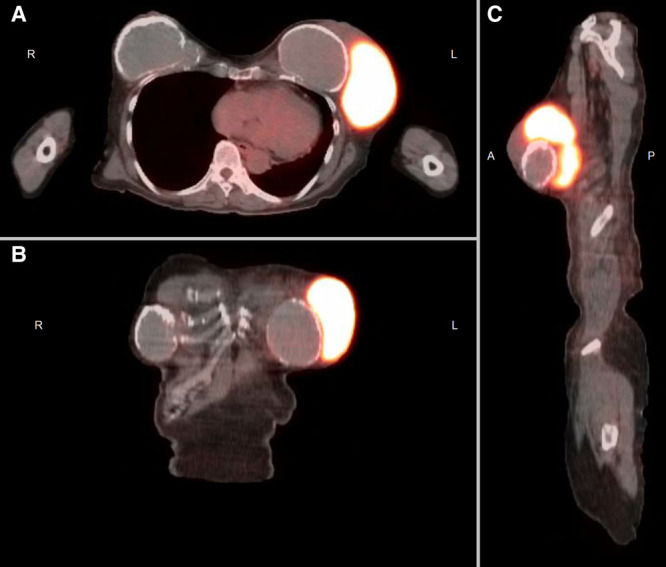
PET-CT imaging demonstrating intensely avid periprosthetic lymphoma mass in the transverse (A), coronal (B) and sagittal (C) planes. PET-CT = positron emission tomography-computed tomograph.
